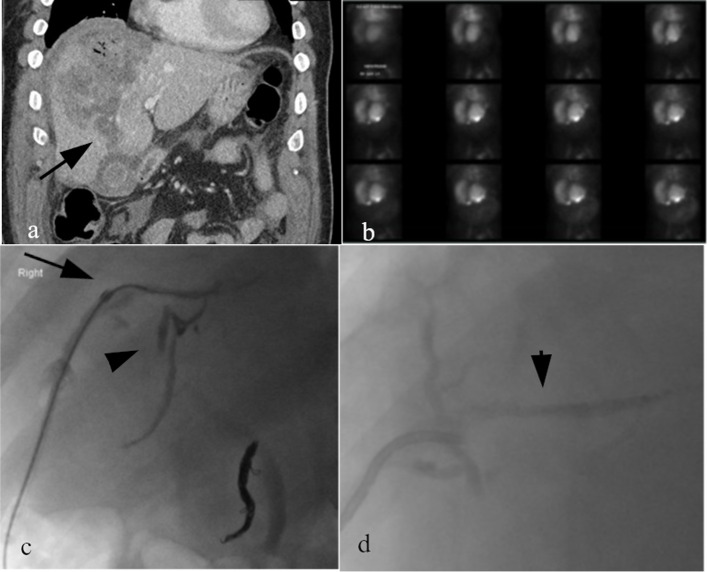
a High grade liver laceration seen on contrast enhanced CT. b HIDA scan with evidence of biliary leak. c Catheterization of hepatic duct (arrow) with contrast injection confirming site of leak (arrowhead). d Post embolization cast in site of biliary leak (arrow)
